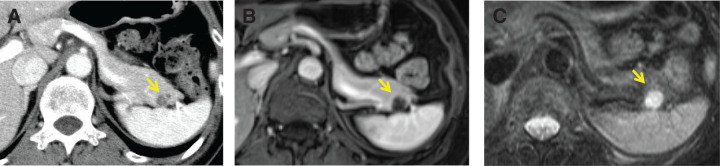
Imaging manifestations of pancreatic lesions (as indicated by the yellow arrow) in Case 1. (A) Abdominal CT shows the tail of the pancreas and the wall of the cyst, which is partially enhanced. (B) MRI reveals a cystic lesion with low signal intensity on T1-weighted images. (C) MRI reveals a cystic lesion with high signal intensity on T2-weighted images.
